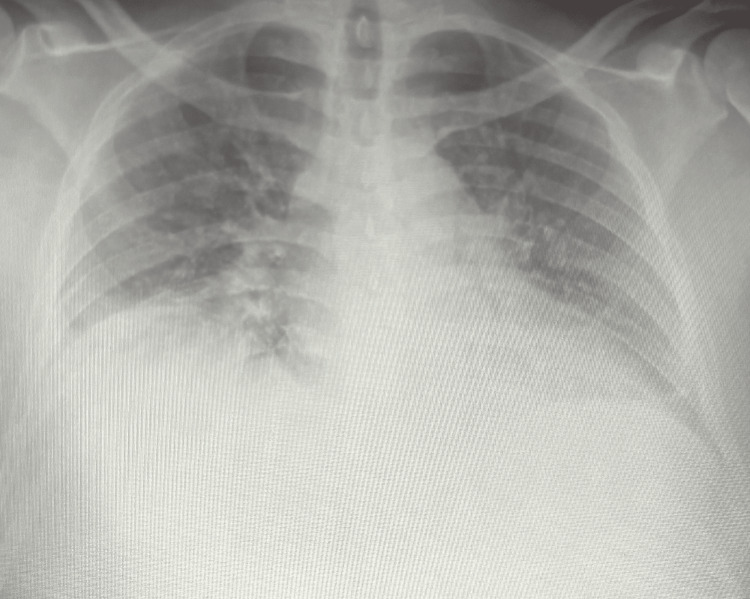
Chest X-ray showing bibasal consolidation
